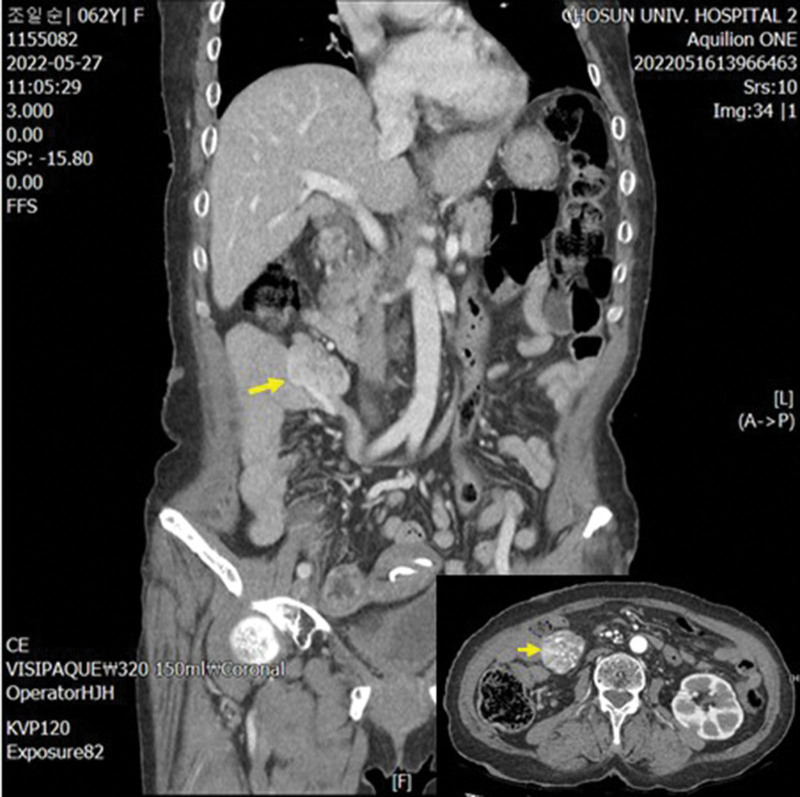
Abdominal and pelvic CT findings. An enhancing mass of approximately 4.1 cm (arrows) was identified near the ileal mesentery. CT = computed tomography.
